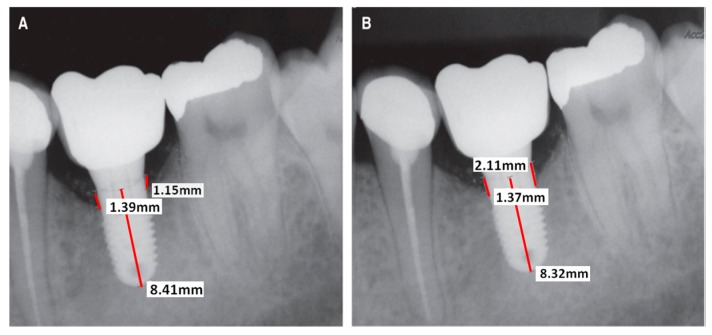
Example of measurement using periapical radiographs. (A) Left radiograph was taken after delivering a prosthesis, (B) Right radiograph was taken during the follow-up period. Both radiographs are Zimmer^®^ commercial implants in the same patient. Figure adapted from Cervino [99].
